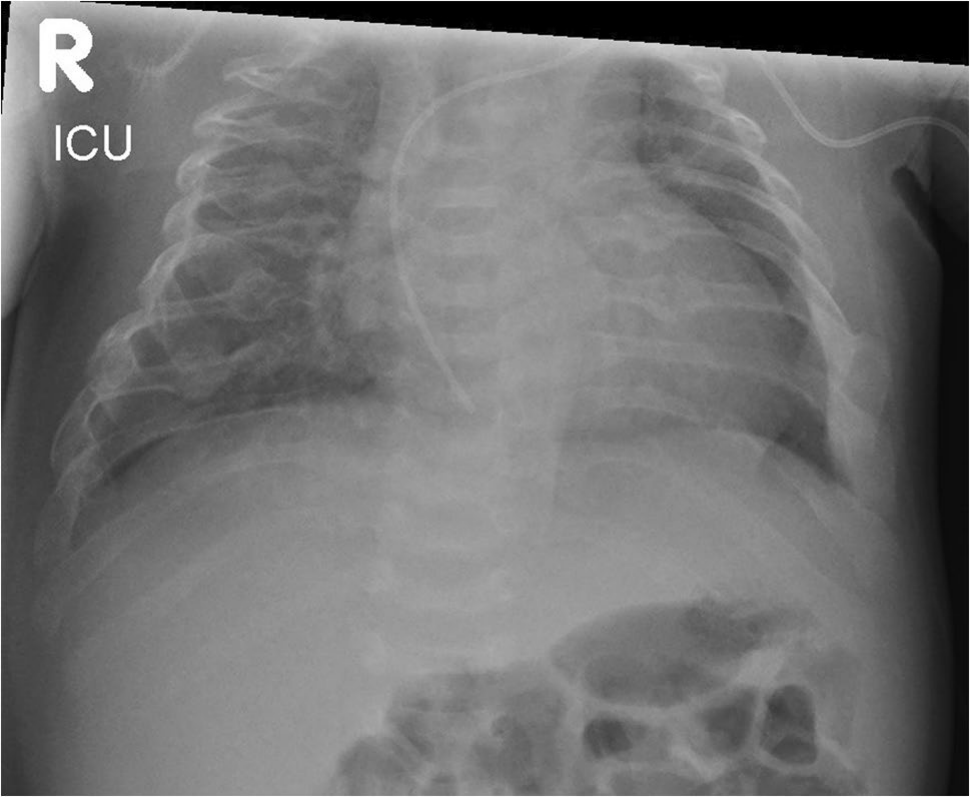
Plain radiograph of chest of an infant with severe osteogenesis imperfecta on a neonatal intensive care unit. There are multiple rib and vertebral fractures with some reduction in chest size. The infant was self-ventilating in low-flow oxygen at the time of the radiograph and did briefly show signs of respiratory failure on blood gas measurements but did not require continuous positive pressure support
